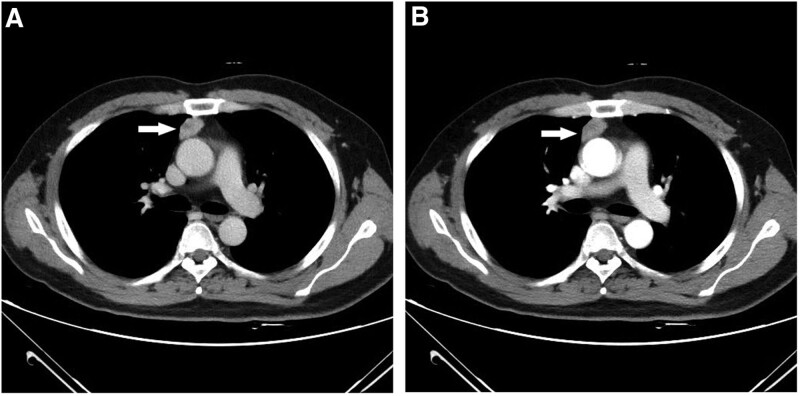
Pre-operative CT scan of the mediastinum. (A–B) the mediastinal CT showing a soft tissue lesion in anterior superior mediastinum with homogenous enhancement. CT = computed tomography.
